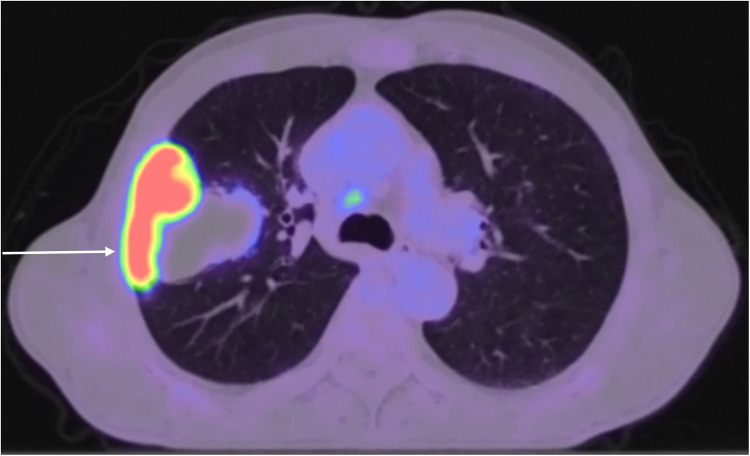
18F-fluorodeoxyglucose positron emission tomography integrated with CT showing heterogeneous radiotracer uptake within a pulmonary mass originating in the right upper lobe and infiltrating the chest wall, as well as low-grade metabolic activity in right lower paratracheal lymph nodes.
